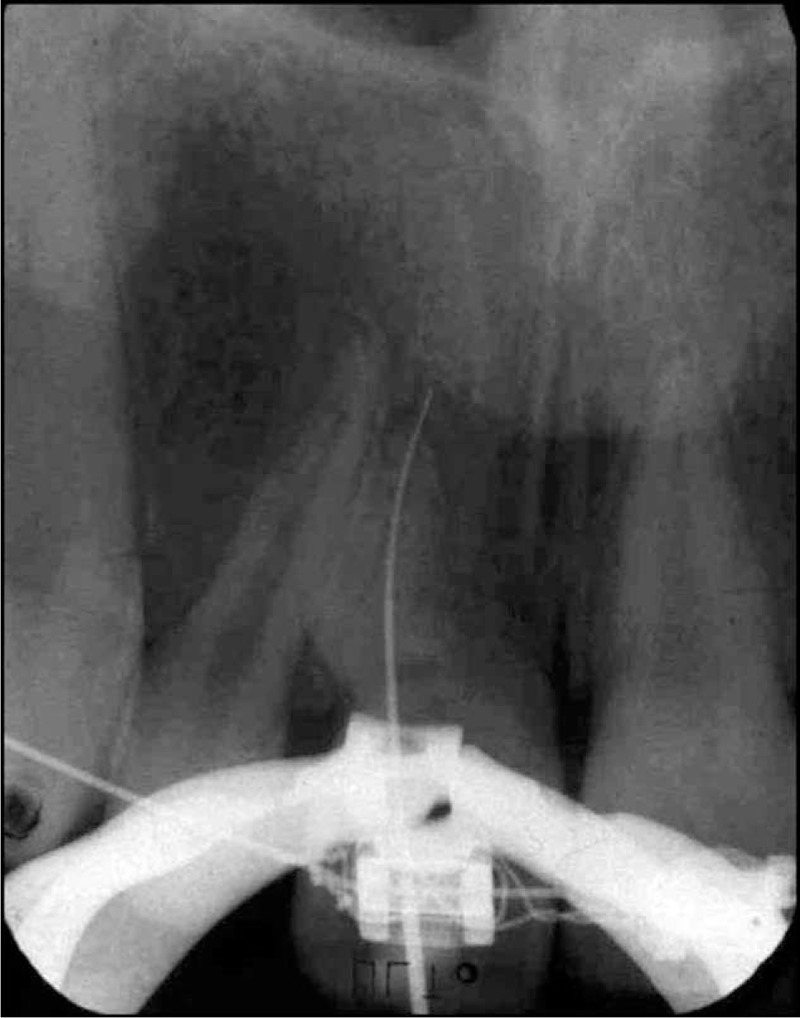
Radiographic measurement of the working length with a Kerr file #15.
